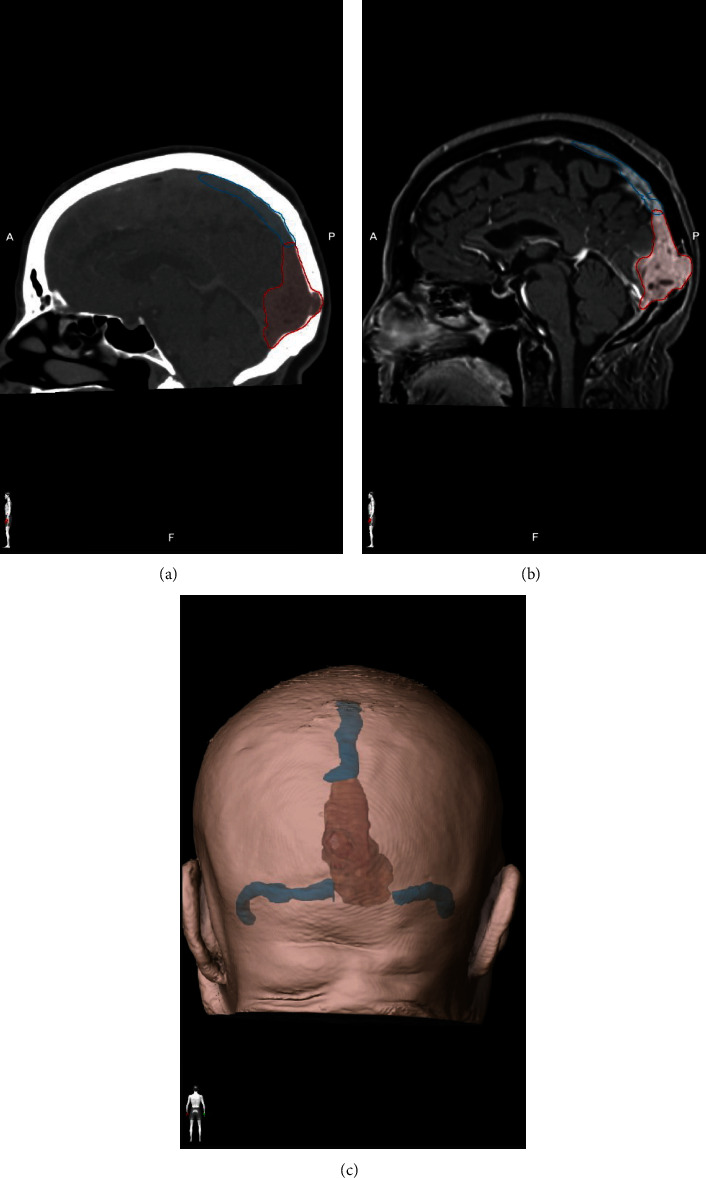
Representative images of intracranial hemangiopericytoma invading the CoS, the SSS, and RTS. (a) CT scan images shows the bony invasion. (b) MRI shows heterogeneously enhancing mass inside the confluent of sinuses. (c) Brainlab^®^ 3D reconstruction of the tumor (red) in relation with dural sinuses (blue).
